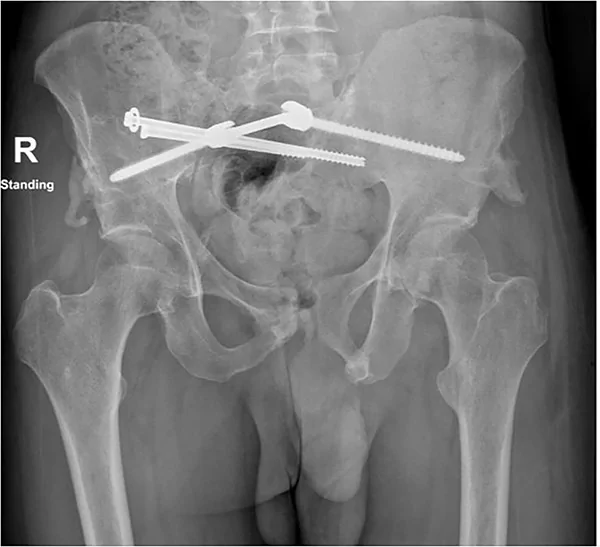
Pelvic radiograph after removal of the INFIX component, with remaining posterior pelvis implant.
